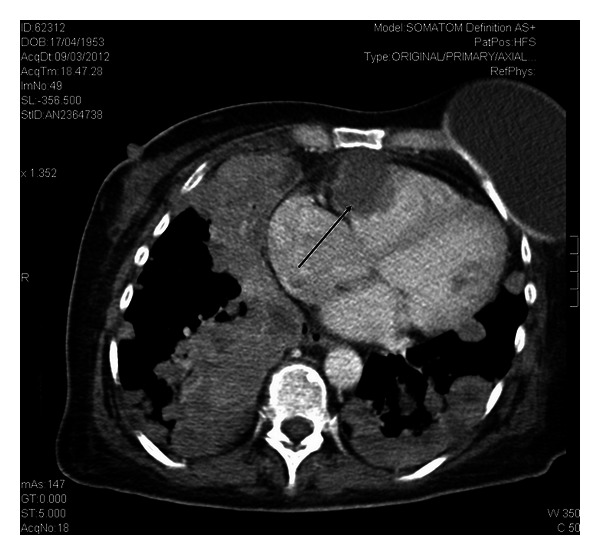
Mass involving right ventricular sections. Chest high-resolution computed tomography pulmonary angiography showing mass pertaining to the pericardium with intracardiac extension at level of anterior right ventricle wall causing occlusion of tricuspid valve (arrow).
